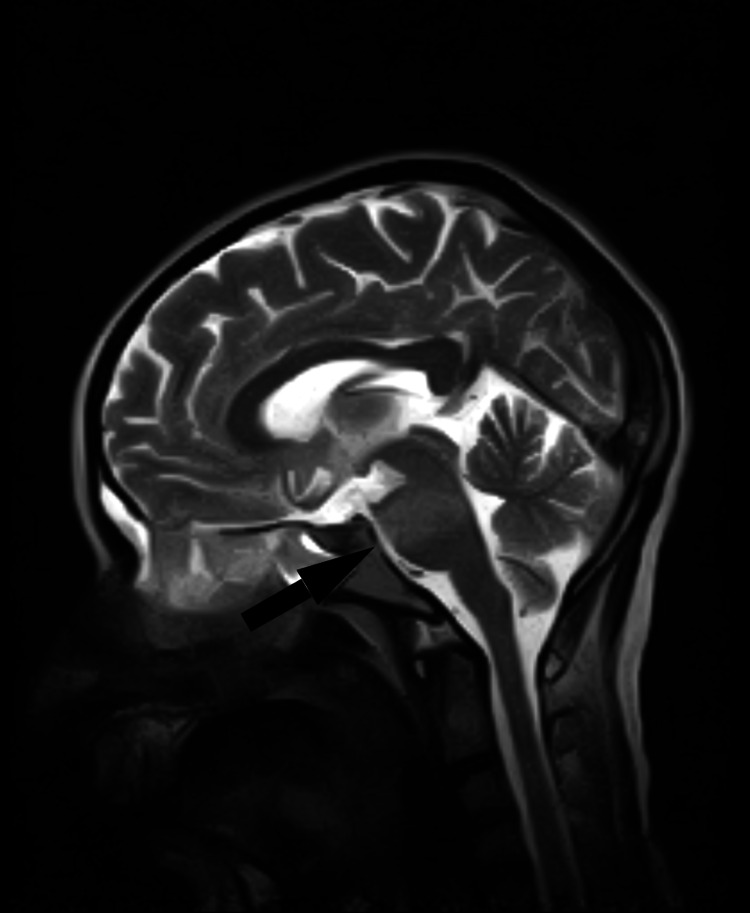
MRI brain, sagittal, T2-weighted image shows a subtle abnormal hyperintense signal in the region of pons (arrow). MRI: magnetic resonance imaging.
